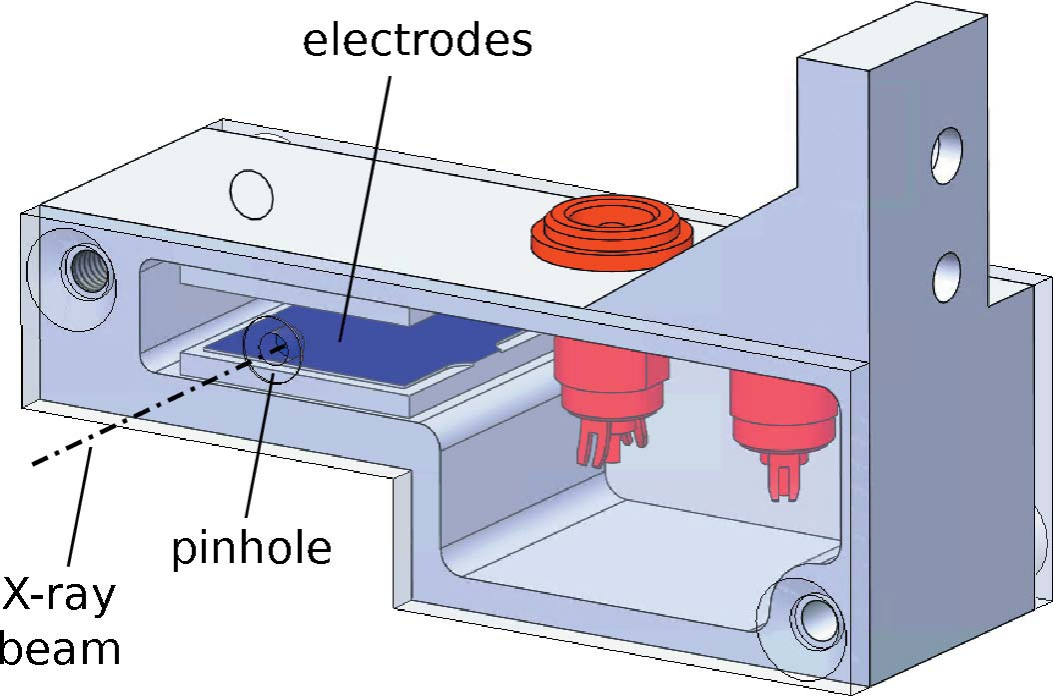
A rendering of the ion chamber. Parallel plate electrodes (blue), a recess for mounting a pinhole (identical recesses are found on both the incoming and outgoing sides) and the outgoing X-ray beam are marked. The electrical connectors are in red and the internal cabling is omitted.
